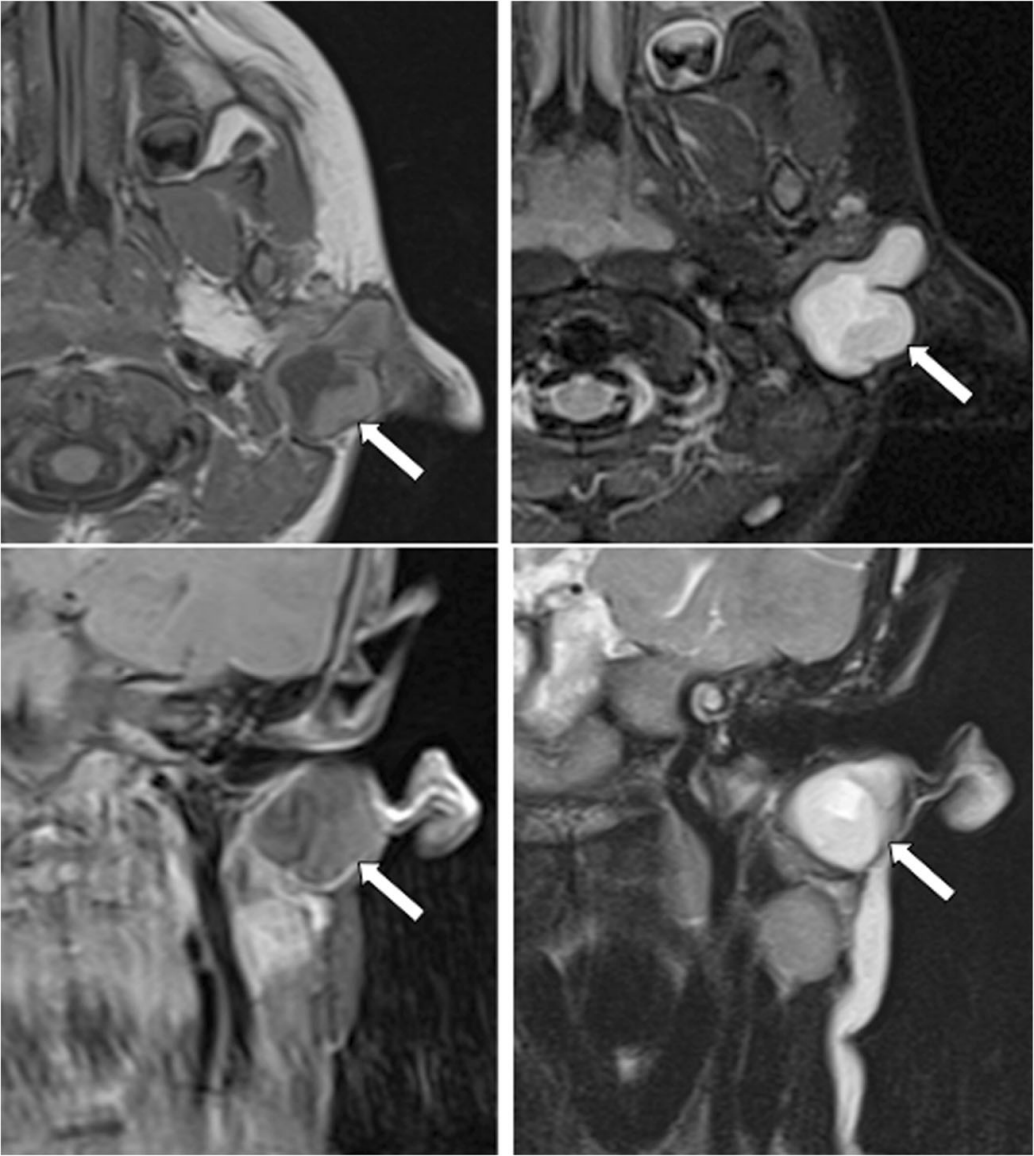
MR imaging from a child presenting with purulent ear discharge. Axial and coronal T1 post-contrast (*left hand images*) with axial and coronal STIR images (*right hand images*) demonstrating a thick-walled sinus tract (*white arrows*) that extended to the clinically apparent opening in the left external auditory canal. The tract was surgically excised and confirmed to represent a first branchial cleft anomaly
